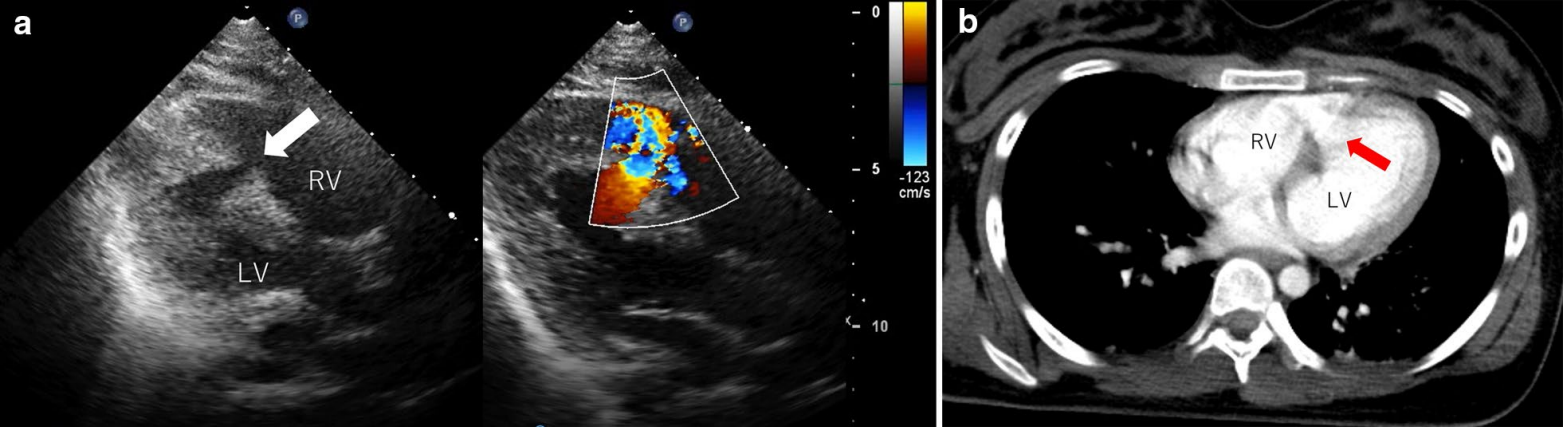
a Echocardiography findings showing ventricular septal rupture (arrow) at the muscular septum, and a left-to-right shunt with a pulmonary-to-systemic flow ratio of 4:1. b Chest computed tomography findings indicating defects in the ventricular septum (arrow). LV: left ventricle, RV: right ventricle
